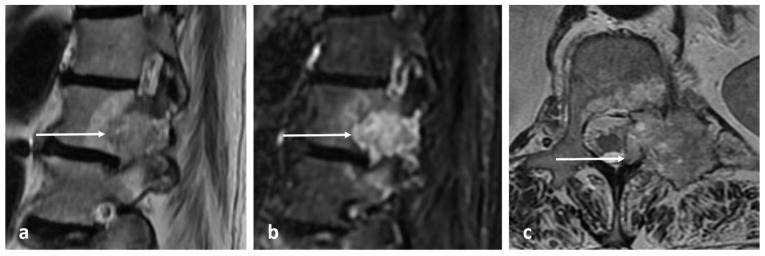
Sagittal T2 (a), STIR (b) and axial T2 (c) images showing an aggressive haemangioma of a thoracic vertebra (white arrows). The osseous destruction and extra osseous soft tissue component is well demonstrated on MRI.
